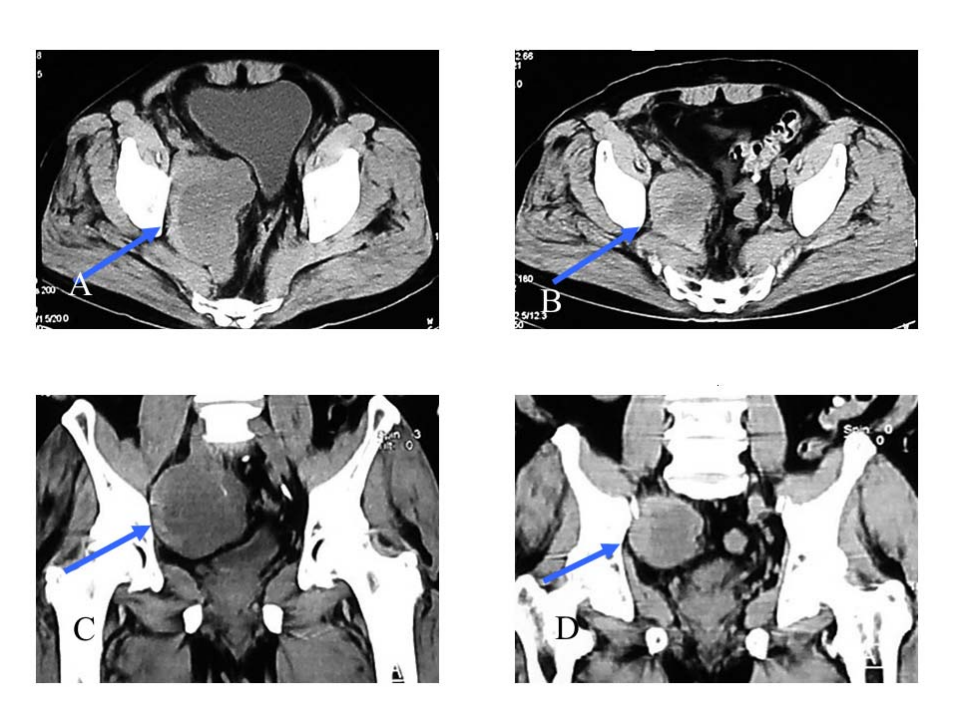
Desmoid tumour before (A, C) and 2 years after (B, D) the commencement of NSAID. Multi planner reformation (MPR)-CT demonstrates the sporadic desmoid tumours originating from the intra-abdominal cavity (arrows). Frontal (A, B) and axial (C, D) images are shown. The tumour has shown a remarkable shrinkage with a regression rate of 68.5% along with disappearance of intratumoural septa.
